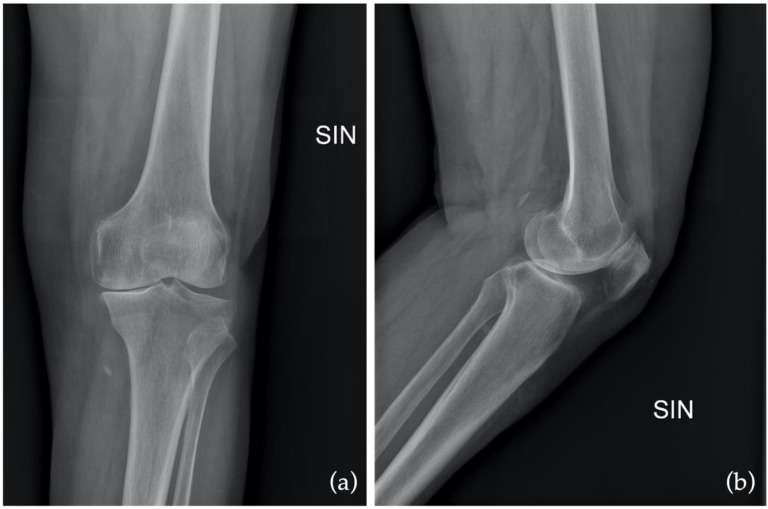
Preoperative X-ray in anteroposterior (AP) as (a) and in latero-lateral (LL) as (b).
